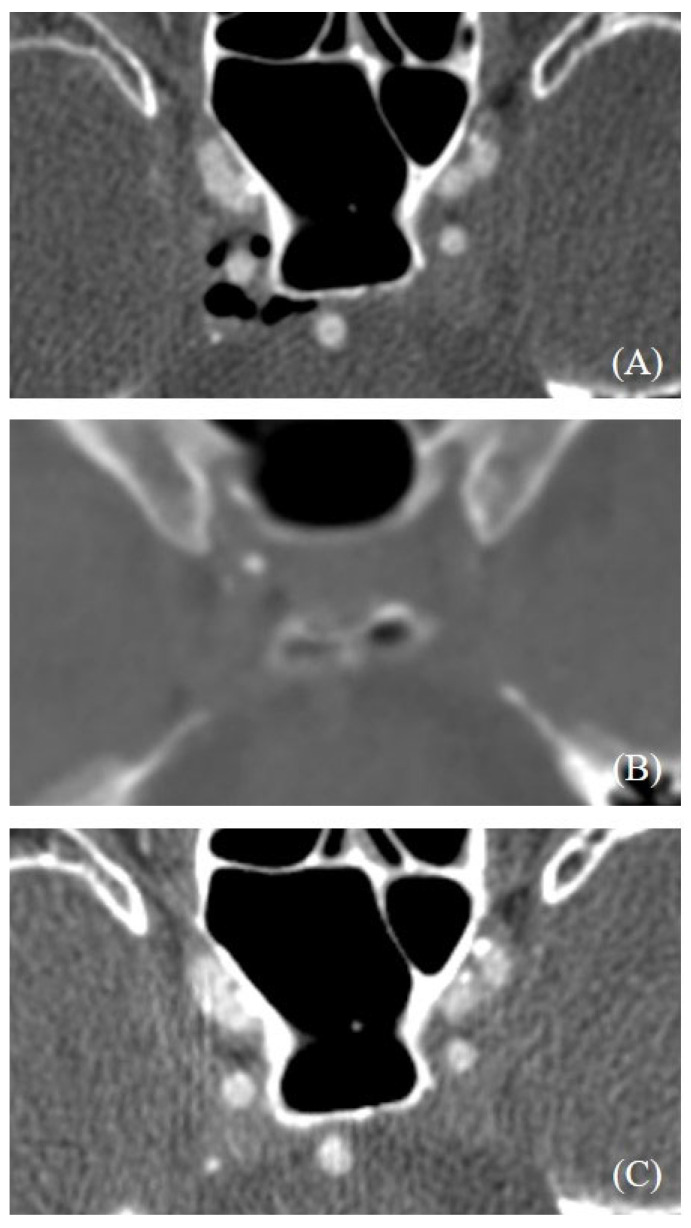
Initial CT angiography showed the cavernous sinus air sign in right cavernous sinus (A). Follow-up brain CT after 1 day (B) and CT angiography performed 7 days after the initial CTA (C) showed spontaneous disappearance of cavernous sinus air sign.
